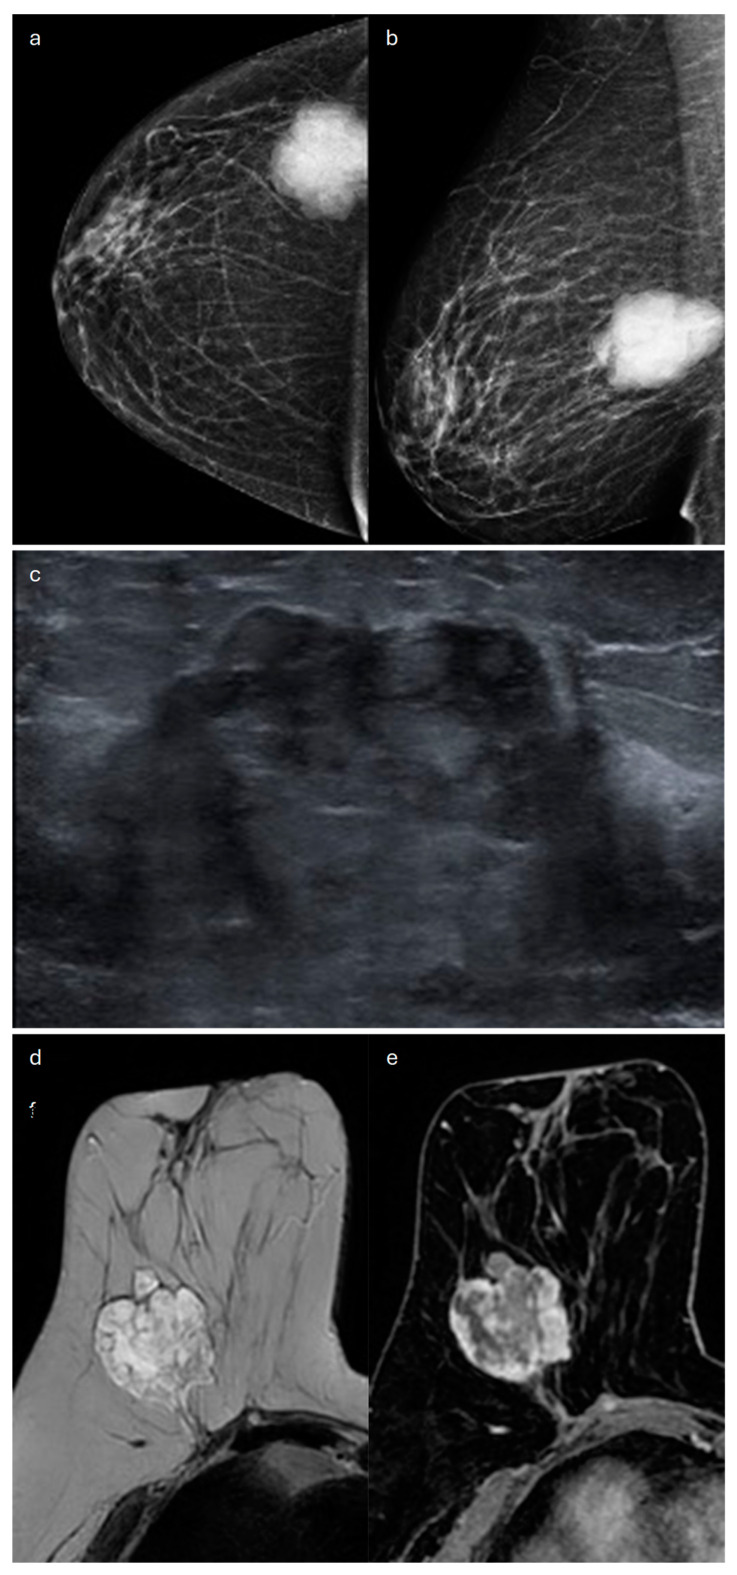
Mucinous carcinoma in a 58-year-old patient presenting with a right-breast lump on clinical examination. (a) Cranio-caudal and (b) medio-lateral oblique mammograms of the right breast show an oval-shaped mass with circumscribed margins at the junction of the upper and lower outer quadrants (c) corresponding to a hypoechoic lesion on ultrasound. (d) Axial T2-weighted MRI image shows multiple areas of high signal intensity within the mass corresponding to the mucinous content. (e) Axial T1-weighted fat-suppressed dynamic contrast-enhanced MRI image demonstrates a heterogeneous internal enhancement.
